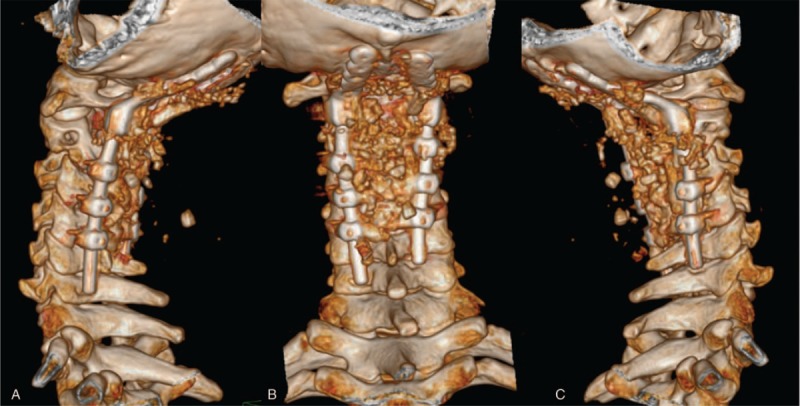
(A and C) After removal of the stitches, 3D-CT was performed and showed that the position of the pedicle screw was not significantly changed from that of the X-ray obtained 2 wk prior. 3D = 3-dimensional, CT = computed tomography.
